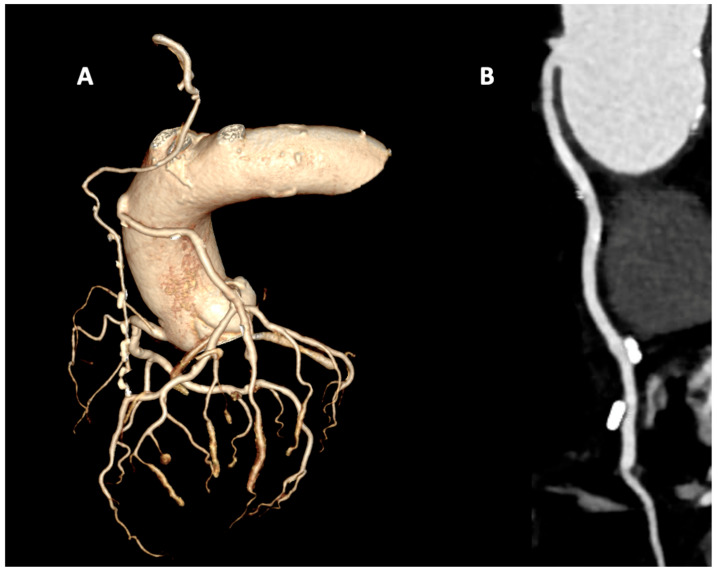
Coronary computed tomography angiography in a coronary artery bypass grafting patient with arterial graft with the left internal mammary artery (LIMA) to the left anterior descending (LAD) artery and venous graft to the intermediate branch. (A) Volume rendering reconstruction with isolated coronary tree that show graft patency. (B) MPR reconstruction of patent venous graft.
